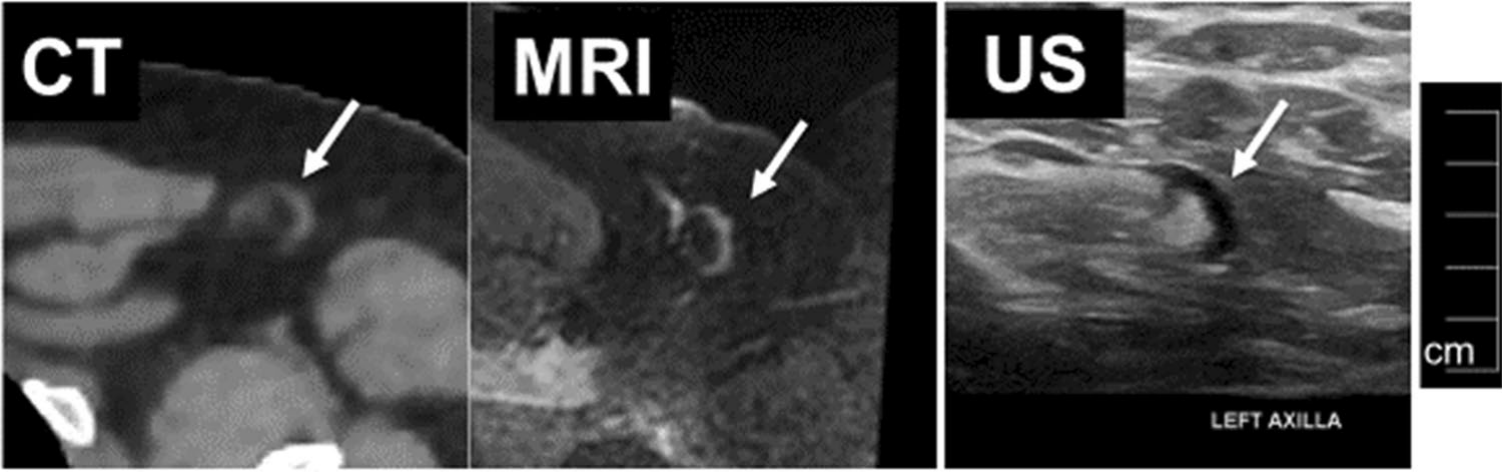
The same normal left axillary node by CT, MRI, and US. All modalities demonstrate the thin cortex (white arrow) and normal fatty hilum
